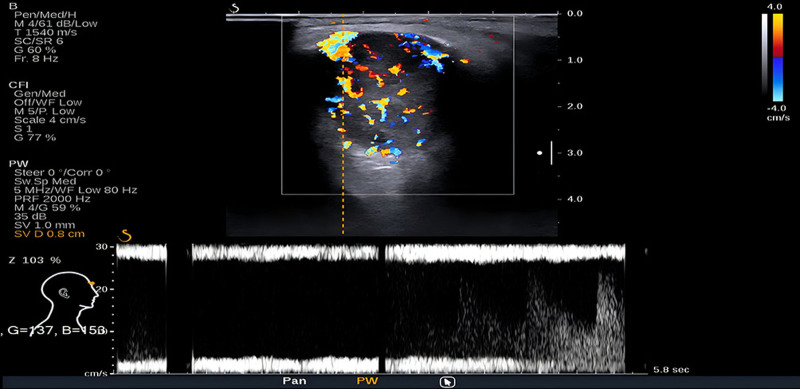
B-type ultrasound showed the size of mass is about 2.6 × 2.6 × 1.5 cm, the CDFI showed abundant blood flow signals. CDFI = color Doppler flow imaging.
